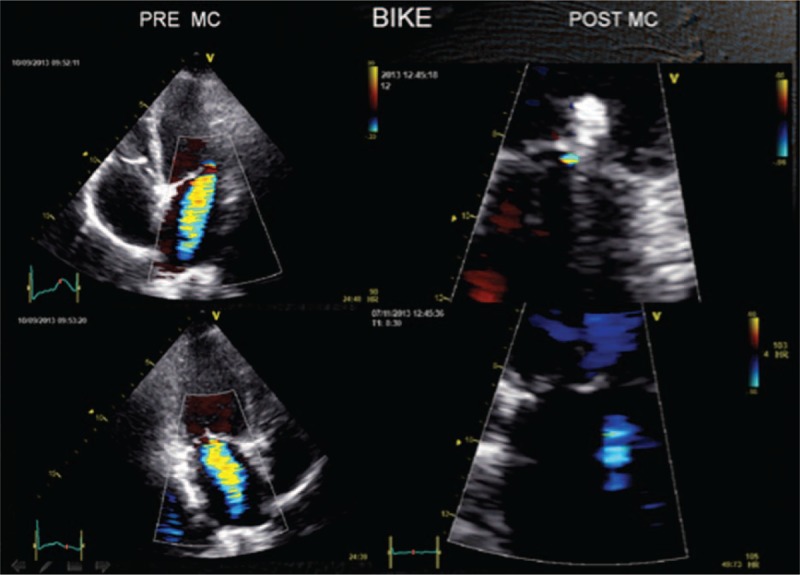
Exercise Doppler echocardiography: on the left, preimplant, moderate-to-severe MR. On the right, postimplant, MR now is negligible. MR = mitral regurgitation.
